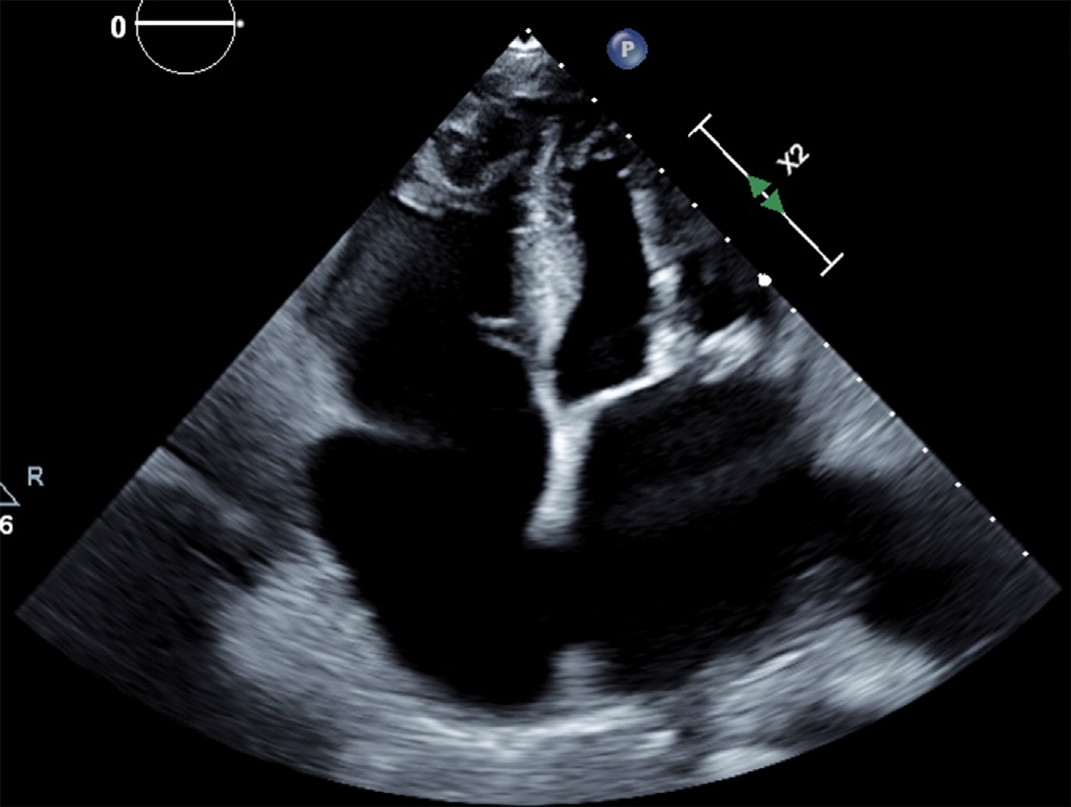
Transthoracic Echocardiogram Apical 4-chamber view demonstrating no pericardial effusion.
